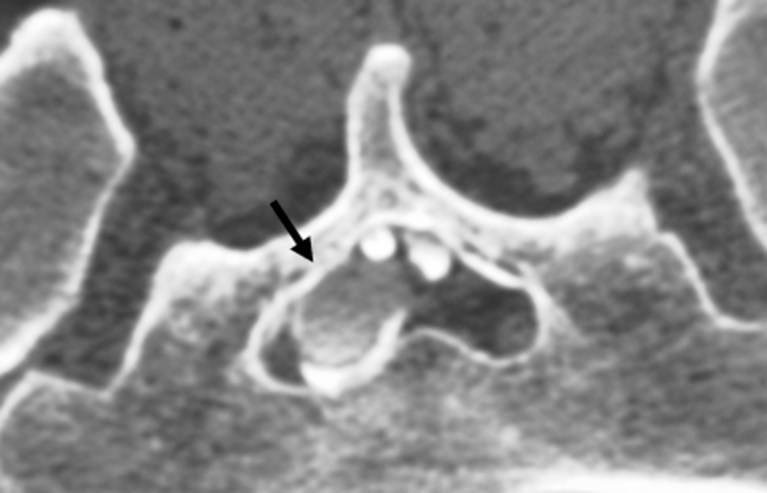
CT myelography in case 8 (prone position, caudal view) reveals the primary cyst (*arrow*). Contrast medium shows only weak filling in the primary cyst
